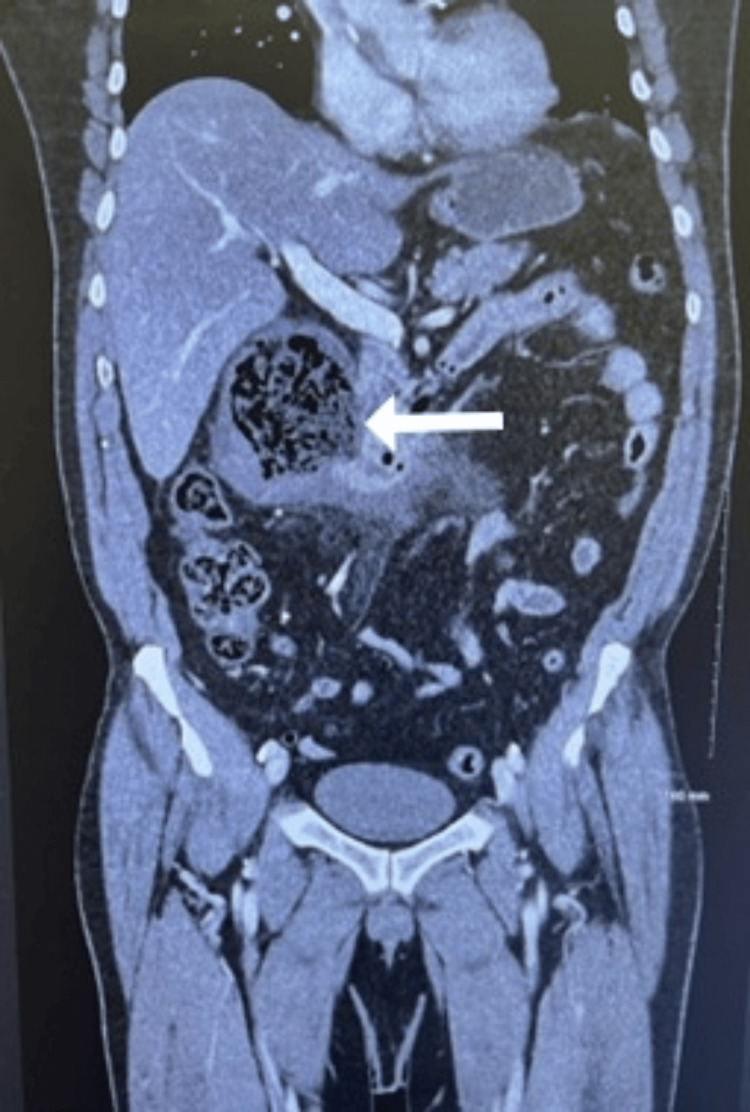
Abdominal CT in coronal view.
